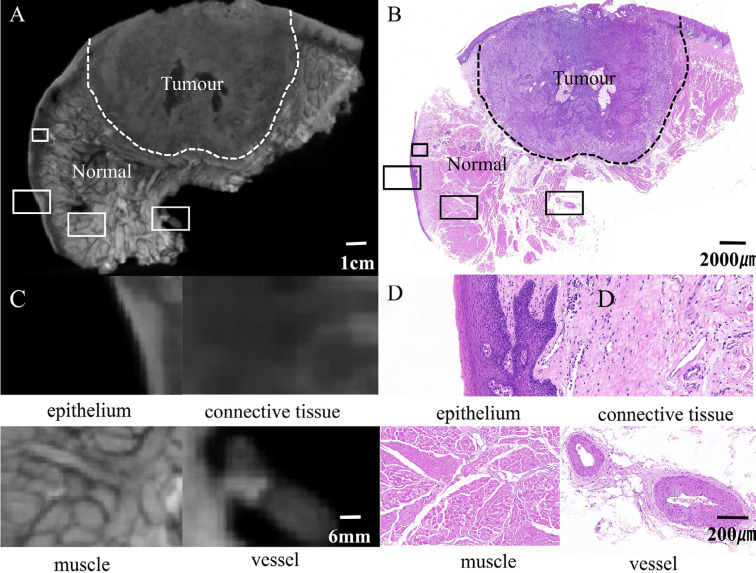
Lugol's iodine-enhanced micro-computed tomography and pathological images of an i*n vitro* sample of oral squamous cell carcinoma. The dashed line represents the tumor boundary. The black boxes correspond to the enlarged parts of the local tissue in the (C) and (D) figures.
